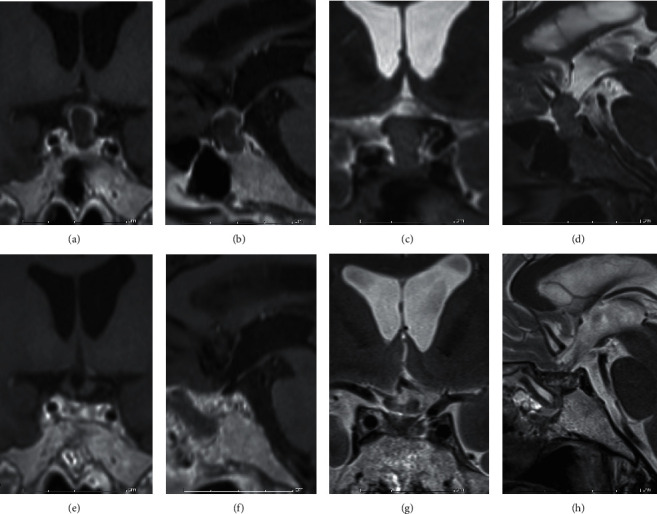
The displaced optic chiasm (a, b, c, and d) is successfully decompressed, and the cyst is totally resected (e, f, g, and h) on MR imaging at 4 months (19 weeks) weeks after surgery.
